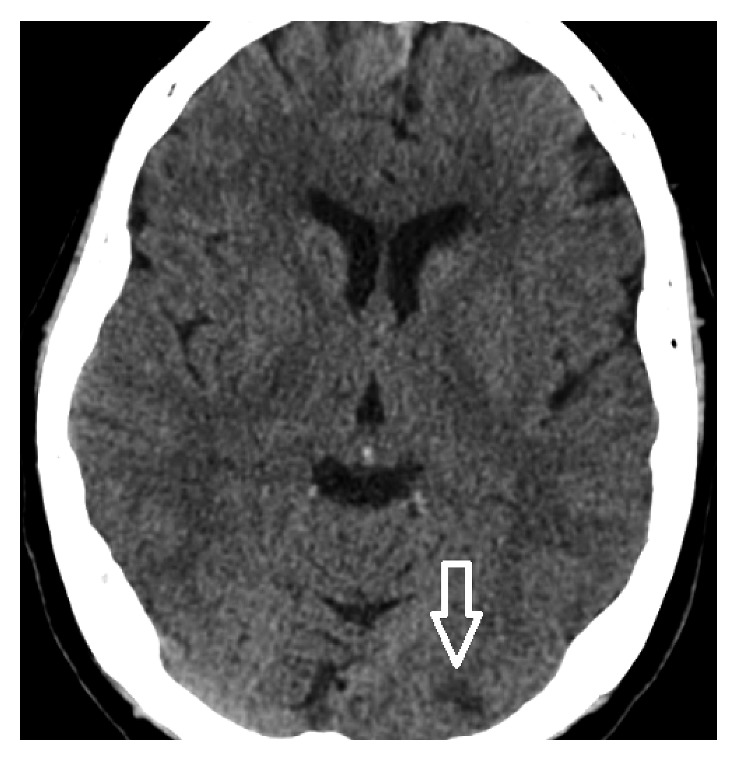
CT scan of the brain without contrast showing an infarct in the left occipital lobe.
